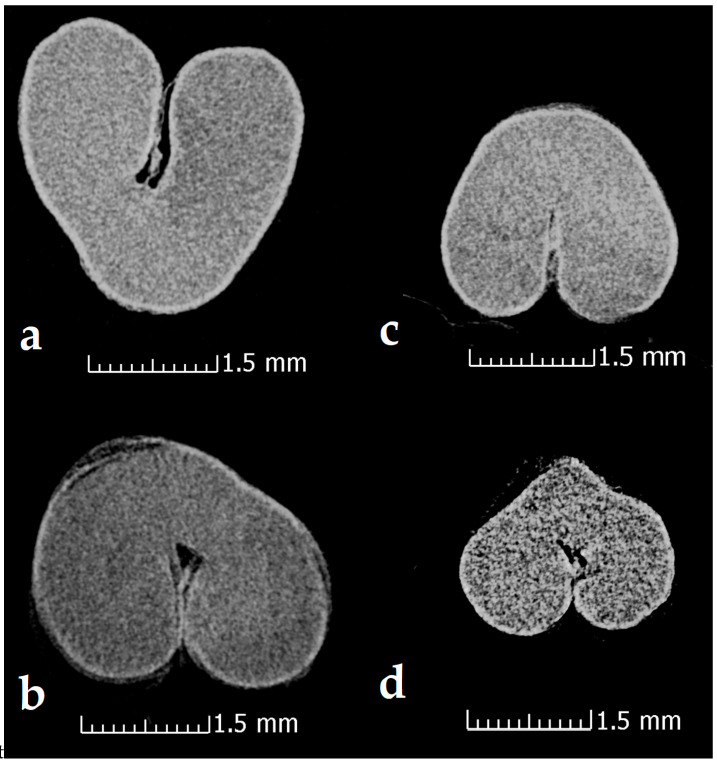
CT image images of control wheat seeds (a,b) (‘Dacic’/’Otilia’) and plasma-treated seeds (c,d) (‘Dacic’/’Otilia’).
